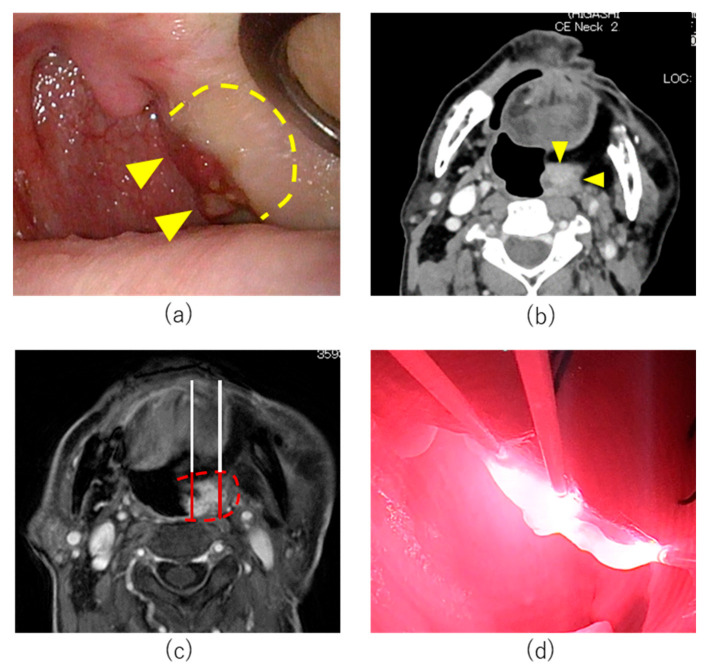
Treatment course of Case 1. (a) Pretreatment endoscopic findings: Left oropharyngeal tumor (arrowheads) and tumor induration (dotted line). (b) Axial section of Gd-enhanced MRI T1 weighted image. (c) Treatment plan of cycle 1 HN-PIT treatment. Planned illumination area (dotted line). (d) Intraoperative image of HN-PIT in Cycle 1. Near-infrared laser was illuminated using 20 mm cylindrical diffusers.
